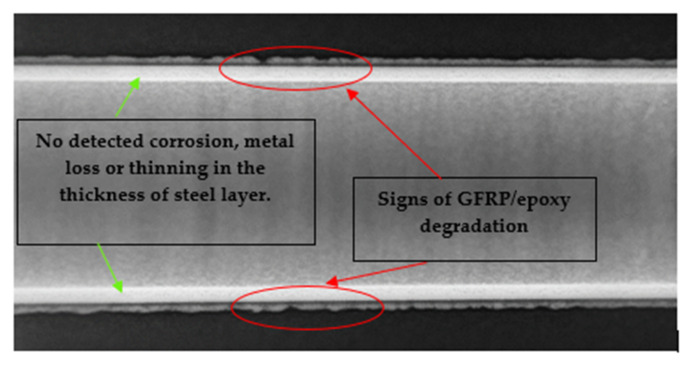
Gamma-ray radiography for the GFRP/steel pipe immersed in HCl solution for 1 year.
